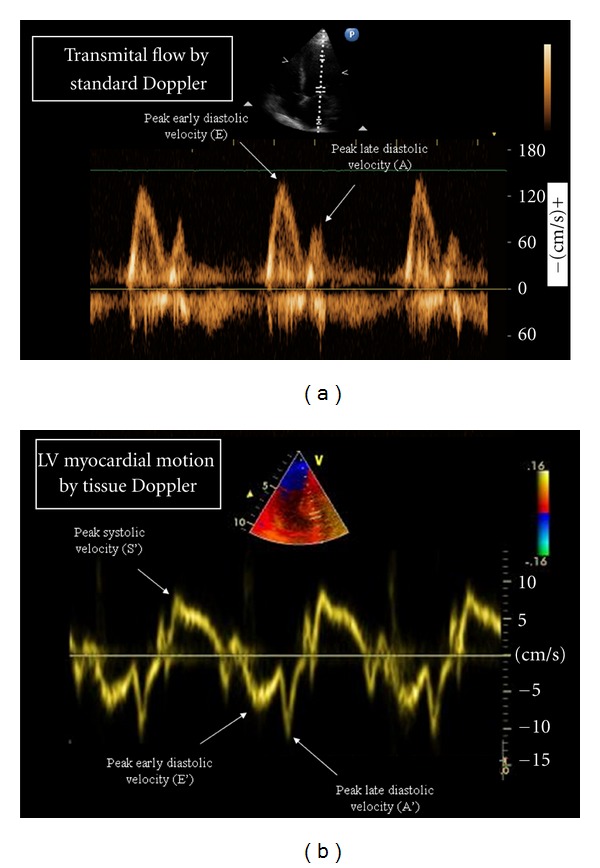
Echocardiographic indexes of left ventricular diastolic function. Standard Doppler of transmitral flow (a) and Tissue Doppler of left ventricular myocardial motion (b). The main indexes for the assessment of left ventricular diastolic function are (1) the peak early diastolic myocardial velocity E′, which is an index of LV relaxation; (2) the ratio between the peak early diastolic velocity of transmitral flow and E′ (E/E′ ratio), which is an index of left ventricular filling pressure.
